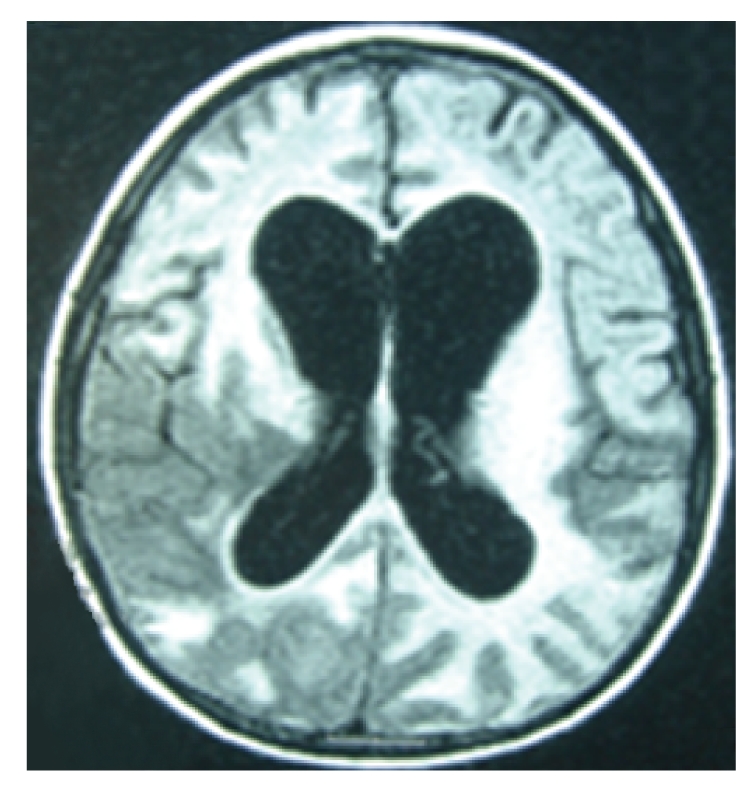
3rd MRI, 4 months after onset of symptoms, 19 days post-HAART cessation.
